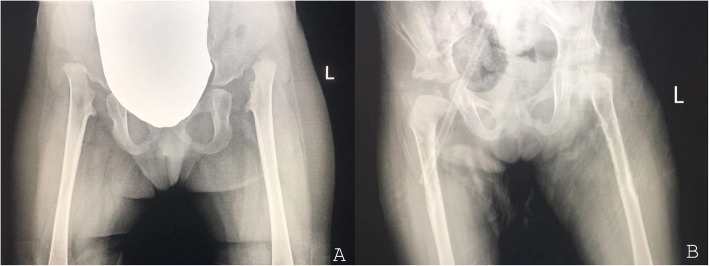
Radiographs of the hip of child 3. a: Hip joint at 3 years old, Swedish key appearance could be observed; b: Hip joint after operation at 4 years old, the postoperative prognosis is good
